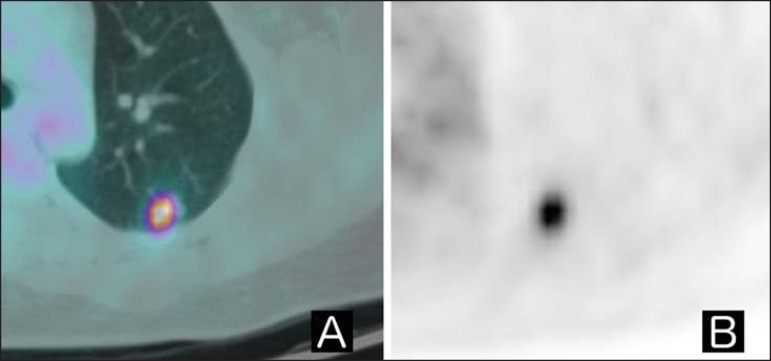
A: Axial fused ^18^F-FDG PET/CT image.
